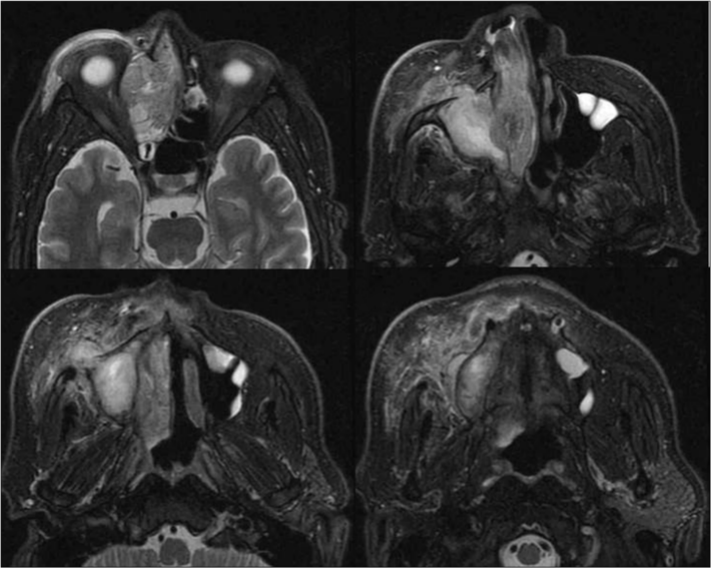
Magnetic resonance imaging scans. Magnetic resonance imaging reveals a tumor mass filling the right nasal cavity and infiltrating the right maxillary sinus, ethmoid sinus, and subcutaneous fat in the right buccal region.
